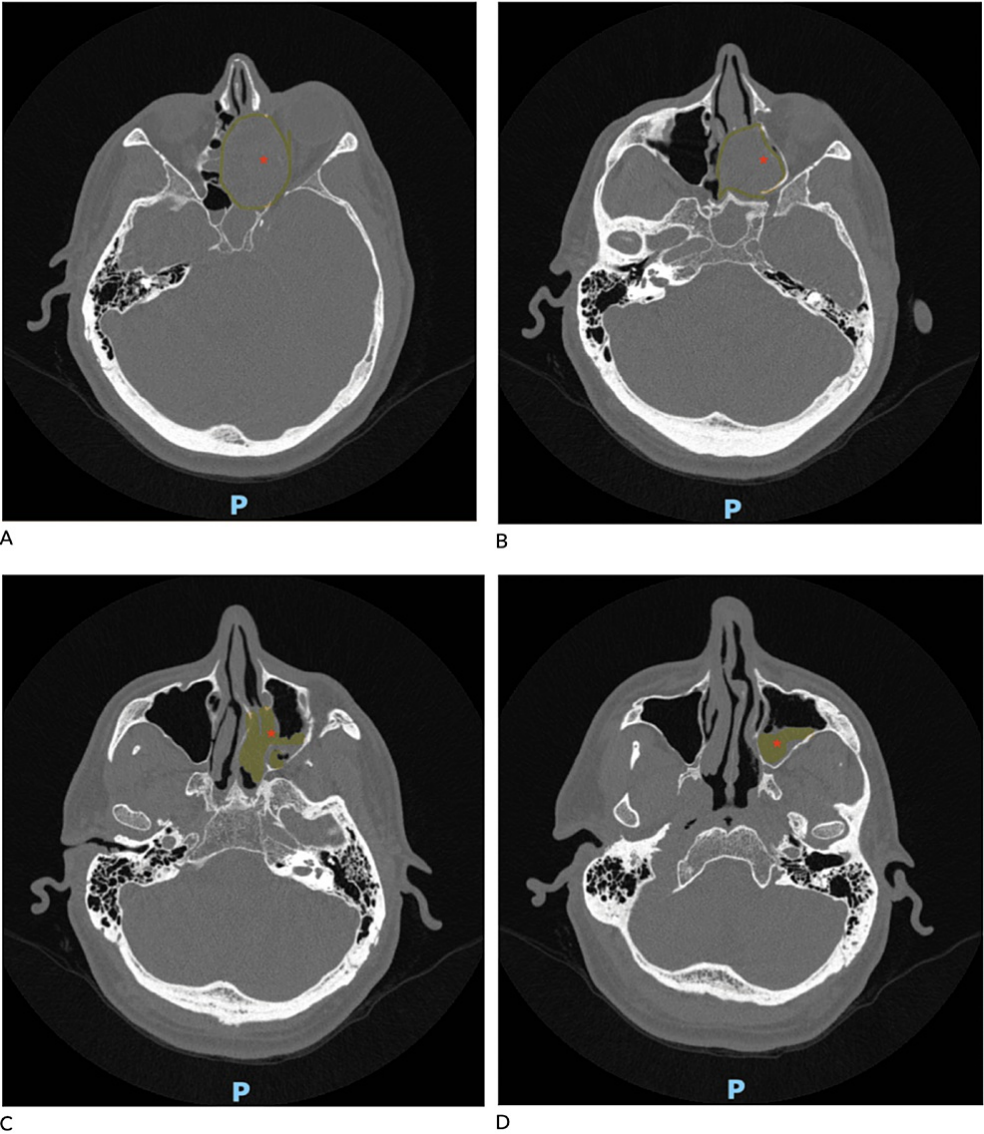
Axial CT sinus landmark without IV contrast from superior to inferior view (A-D). The sinonasal mass is marked with a red asterisk. Yellow shading and outlines indicate orbital and ethmoid sinus invasion of the tumor.
